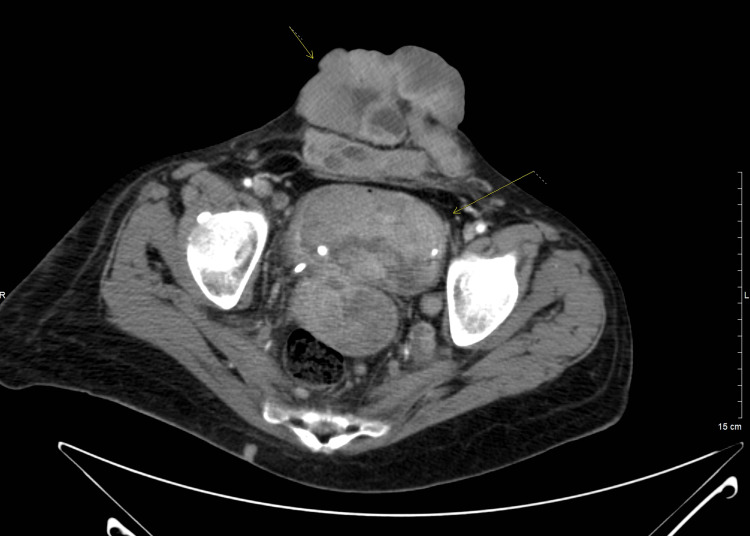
Contrast-enhanced CT of the abdomen and pelvis (axial view) demonstrating a large bladder mass (bottom arrow) involving the bladder with peritoneal metastasis (top arrow).
